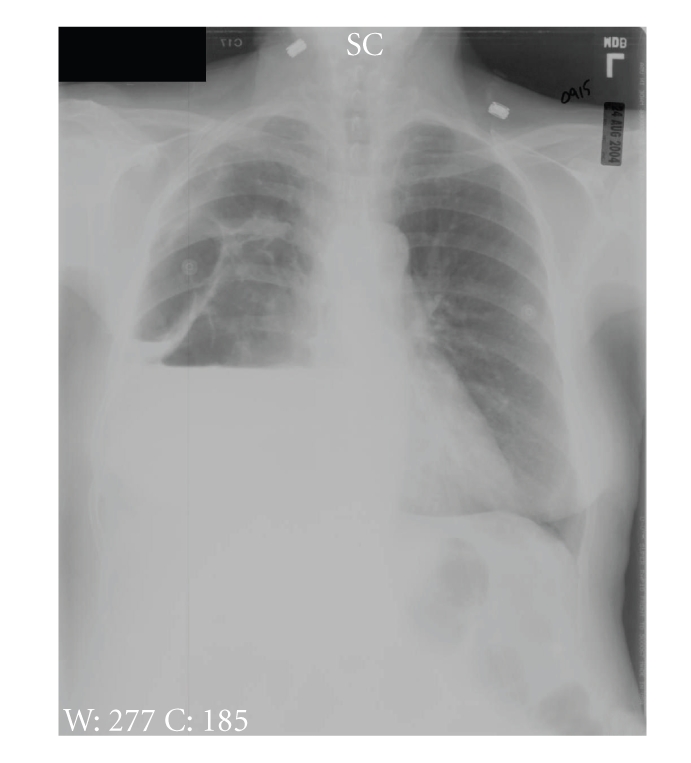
PA chest radiograph from the patient's readmission to the hospital. There is a large right pleural effusion, with an air- and fluid-filled structure noted laterally.
